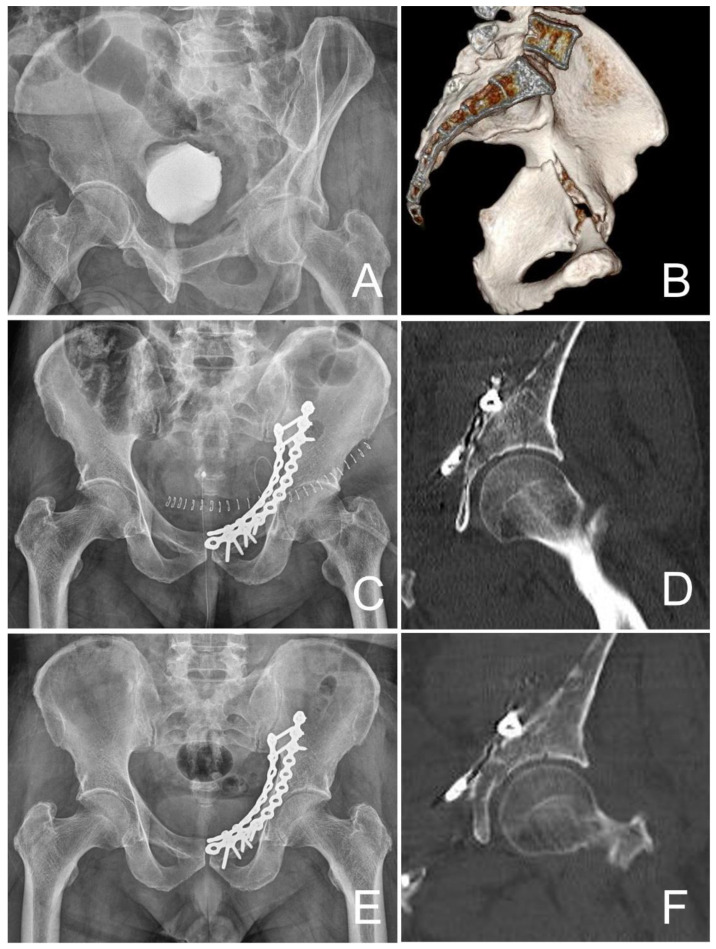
A 66-year-old man underwent direct fixation with infrapectineal plate. (A) Radiograph shows both-column fracture with 13 mm displacement. (B) Three-dimensional computed tomography (CT) scan shows both-column fracture with quadrilateral plate displacement. (C) Direct reduction and fixation with infrapectineal plate is performed. (D) CT scan shows anatomical reduction. (E) Radiograph obtained 24 months after surgery shows union at the fracture site and excellent grade with Matta’s outcome grading. (F) CT scan also shows no sign of osteophyte, joint space narrowing, subchondral sclerosis, and femoral head collapse. CT = computed tomography.
